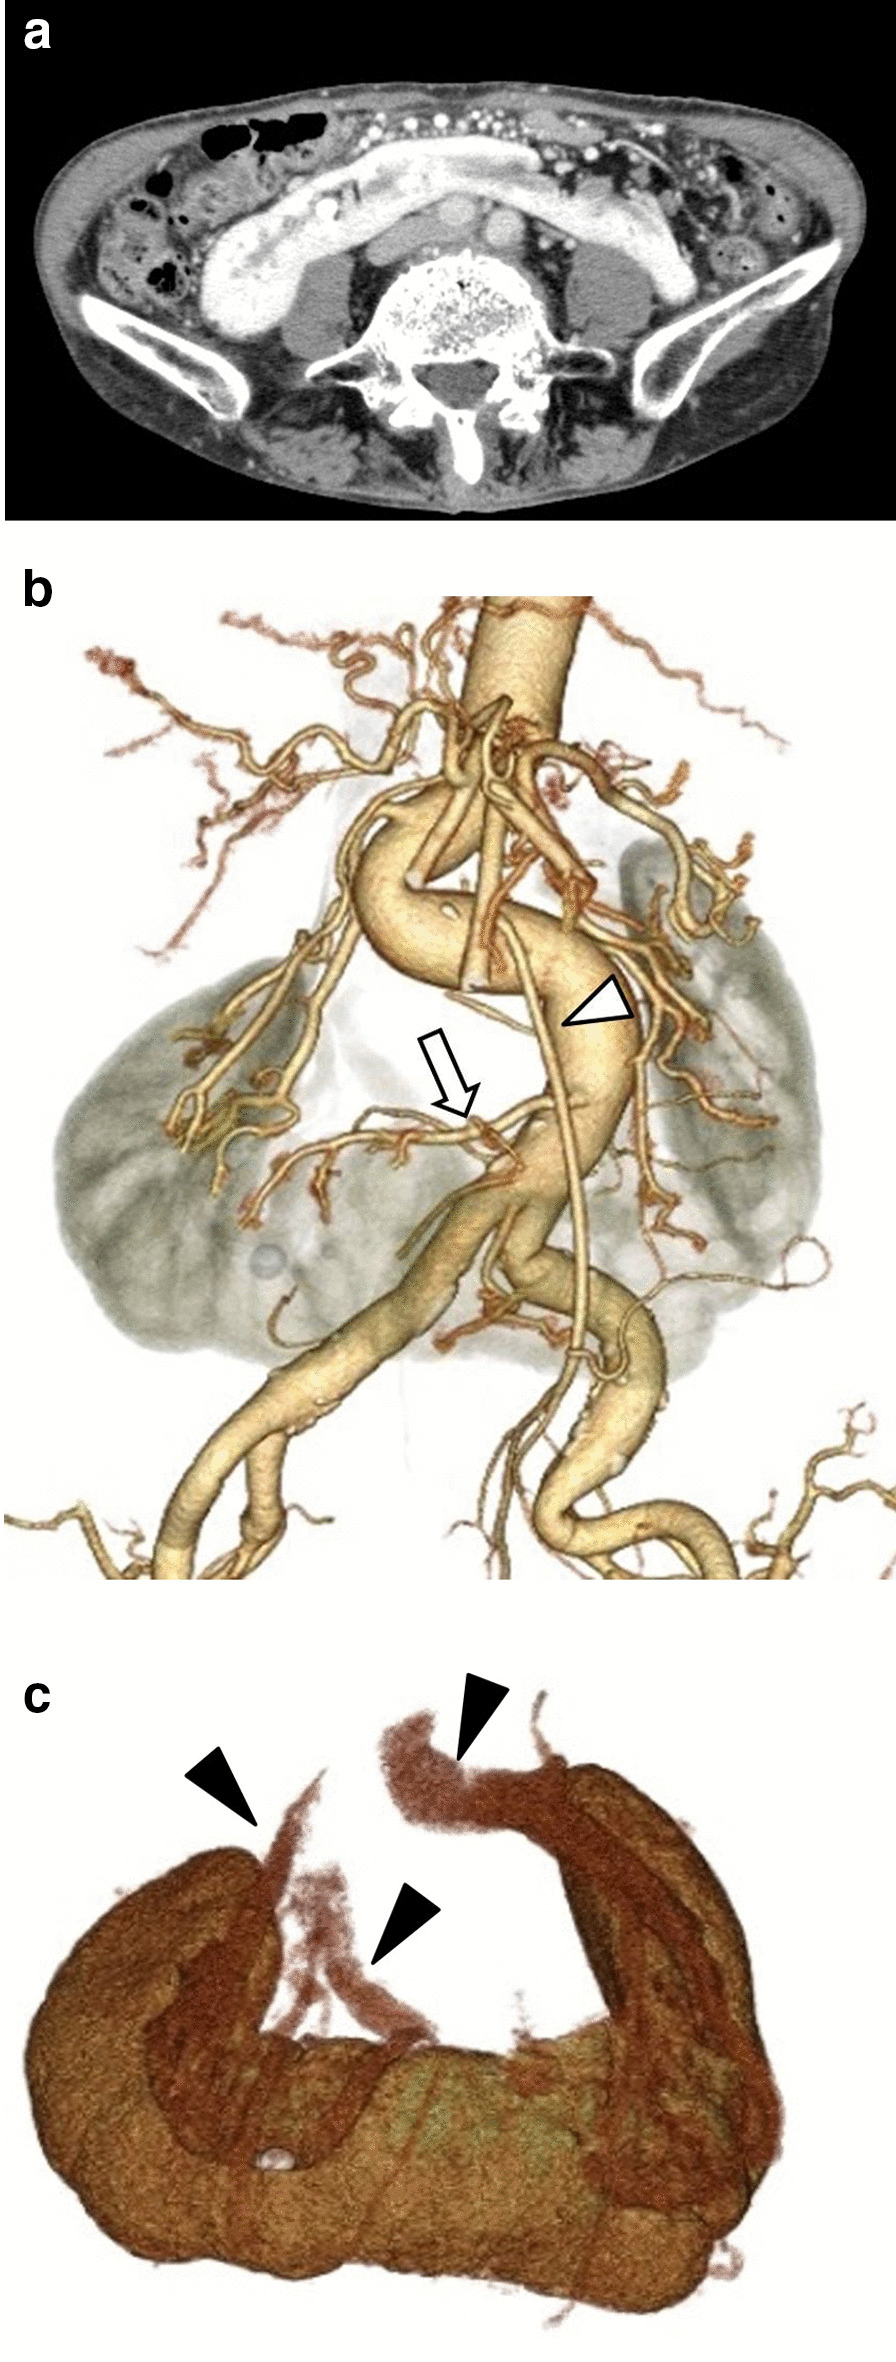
Computed tomography (CT) findings. a Abdominal enhanced CT showing the horseshoe kidney. b, c Three-dimensional CT (3D-CT) angiography showing an aberrant renal artery (arrow) from the aorta below the inferior mesenteric artery (white arrowhead) to the isthmus of the horseshoe kidney and a renal vein from (black arrowhead) the horseshoe kidney
